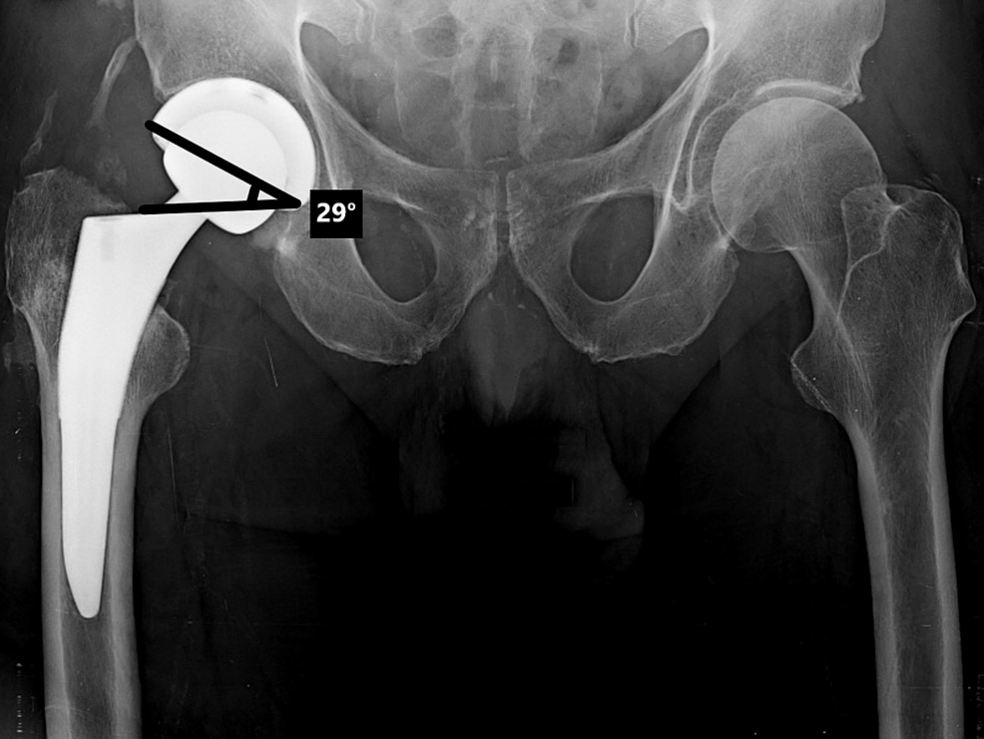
AP radiograph in a patient with recurrent instability. The cup inclination of 29 degrees is outside the so-called safe zone.
